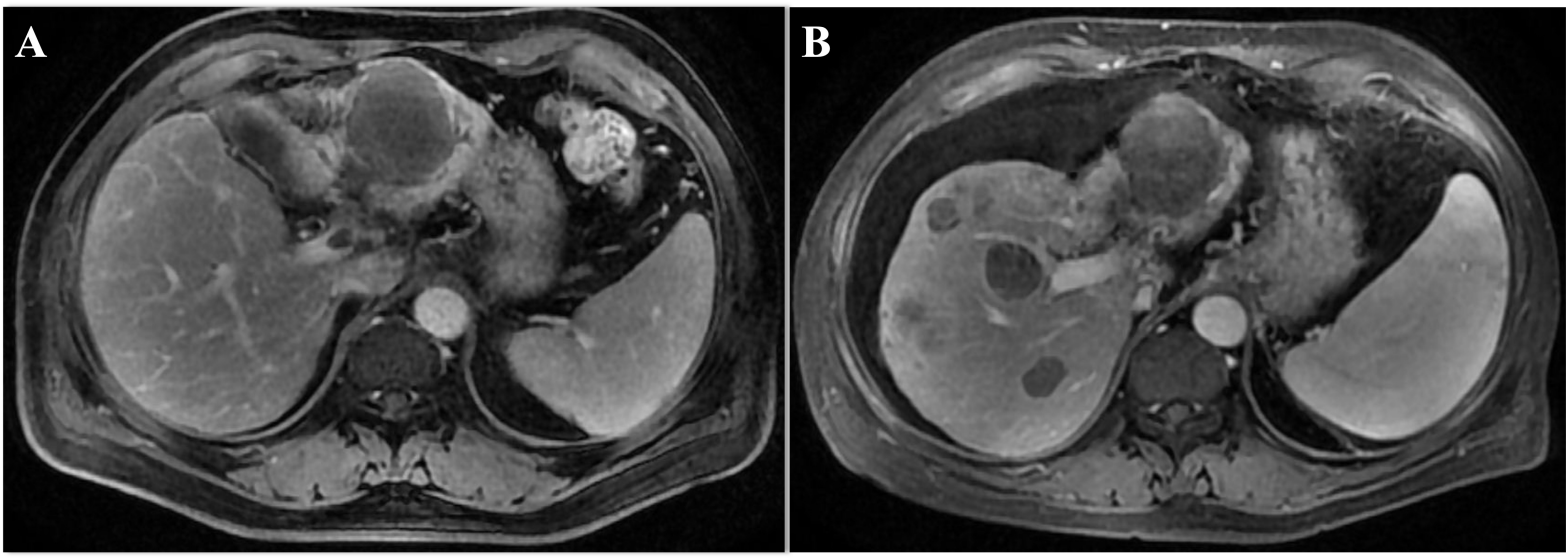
The image of the case during the initial treatment. (A) The original tumor size of liver shrinked significantly 1 week after HAIC and anti-PD-L1 treatment. (B) The enlarged primary tumor of the left lobe of liver and the new lesion appeared in the right lobe after Lenvatinib and anti-PD-L1 treatment. MRI, magnetic resonance imaging.
